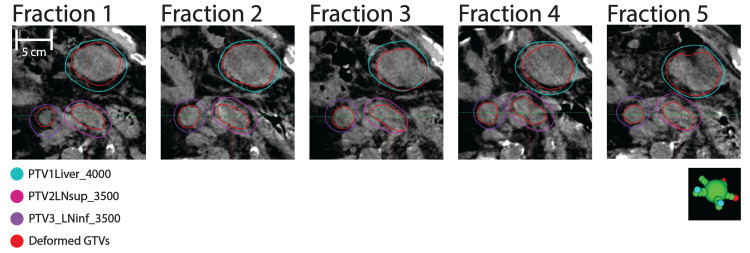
Post-treatment CBCT imaging Comparison of clinical target volume (CTV) and gross tumor volume (GTV) contours (red) deformably mapped onto post-treatment cone beam computed tomography (CBCT) imaging for each fraction relative to PTV contours rigidly mapped based on couch position.
